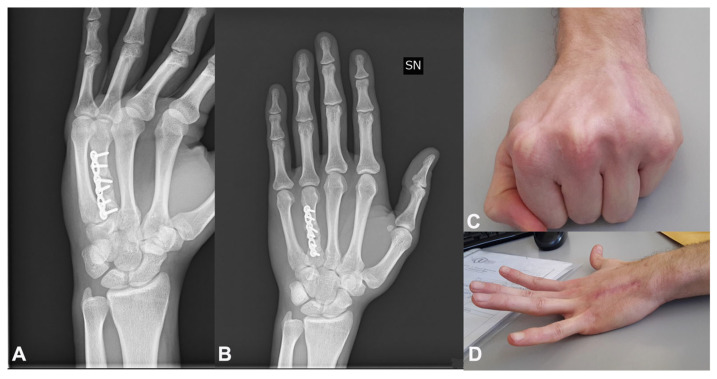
Clinical and radiographic outcome at follow-up: (A,B) Radiographs at 2 months postoperatively demonstrate complete bone consolidation of the fourth metacarpal with restoration of cortical continuity. (C,D) At 3 months, the patient exhibits full functional recovery with excellent TAM, grip strength, pinch strength, and minimal pain. No complications are observed.
